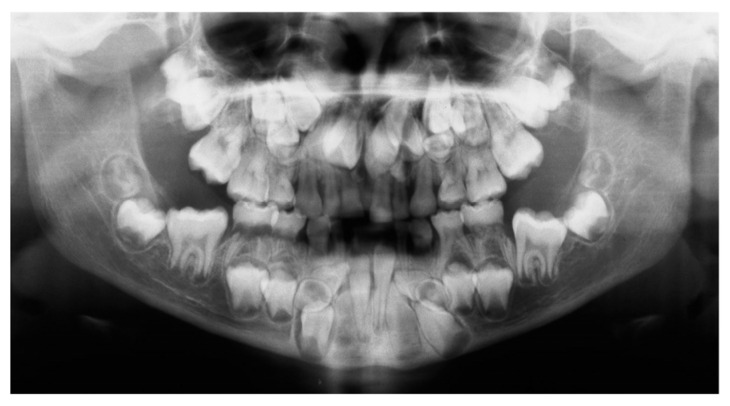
Orthopantomogram of a nearly 10-year-old female patient with Cleidocranial dysplasia. Dentitio tarda is present, the first molars (six-year molars) are not yet fully erupted. Supernumerary permanent teeth are present in the maxilla and in the mandible.
